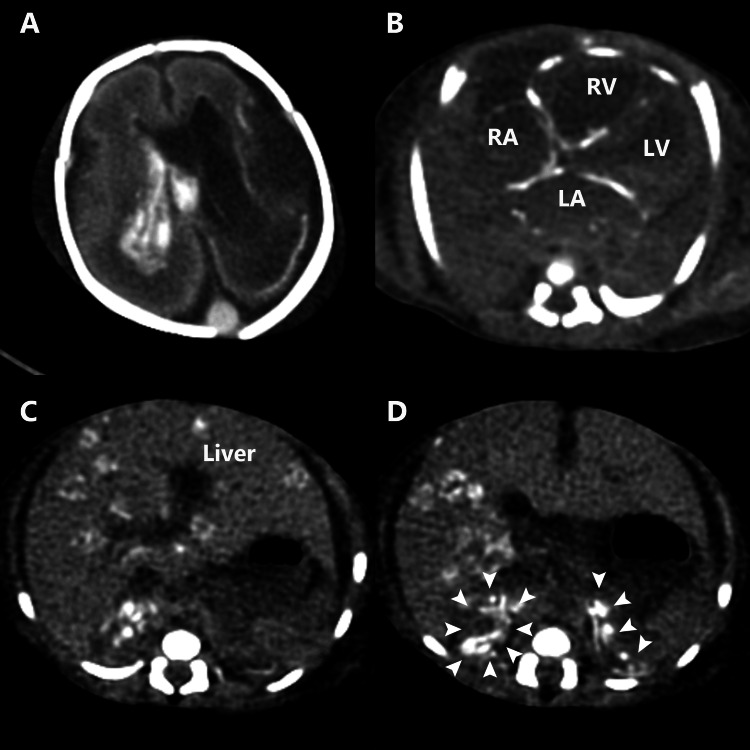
Postmortem computed tomography images. (A) Brain: the right lateral ventricle is dilated, and intraventricular hemorrhage is seen in the left lateral and third ventricles. (B) Heart: hyperdense areas are seen in the heart walls of the atria, right ventricle, and intraventricular septum. (C) Liver: hyperdense round areas are found all over the liver. (D) Adrenal glands: extensive hyperdense areas are found in the bilateral adrenal glands (arrowheads). LA, left atrium; LV, left ventricle; RA, right atrium; RV, right ventricle.
